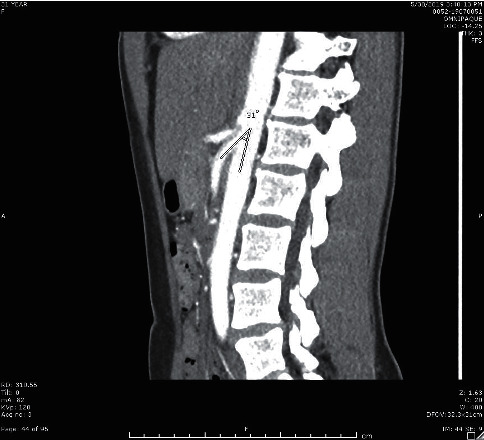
Sagittal image from renal CT with contrast showing decreased angle between aorta and superior mesenteric artery.
